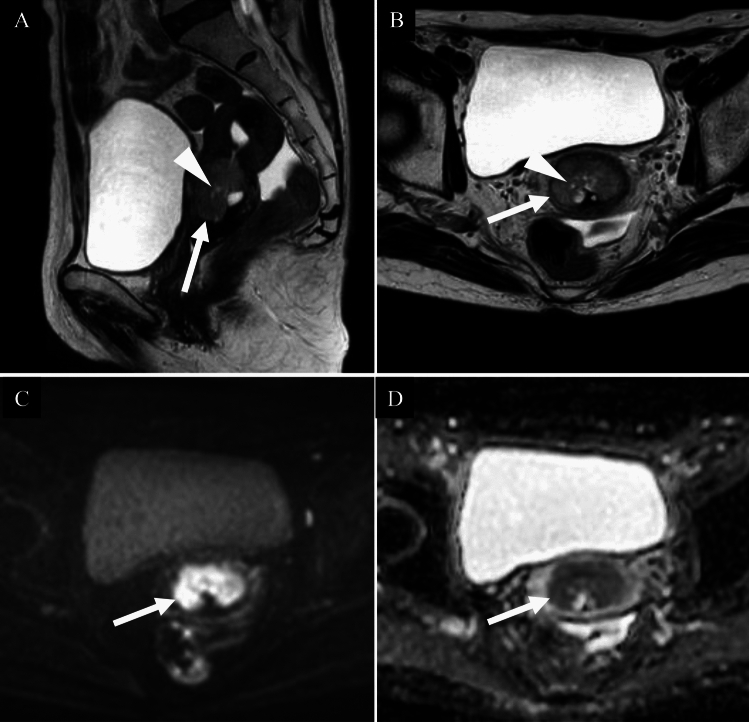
Gastric type adenocarcinoma of the uterine cervix in a woman in her sixties. A Sagittal T2-weighted image; B Axial T2-weighted image; C Axial diffusion-weighted image; D Axial apparent diffusion coefficient map. A T2-hyperintense mass is centered on the anterior wall of the uterine cervix and accompanied by small cysts (A, B: arrowheads) suggestive of gastric type adenocarcinoma (A, B: arrows). The lesion exhibits expansile growth with ill-defined margins. Although the mass shows a high signal intensity on diffusion-weighted image (C: arrow), the reduction in apparent diffusion coefficient values (D: arrow, ADC = 1.116 × 10⁻^3^ mm^2^/s) is less pronounced than that typically observed in squamous cell carcinoma, further supporting the diagnosis. The tumor was pathologically confirmed as FIGO 2018 stage IIB gastric type HPV-independent adenocarcinoma
